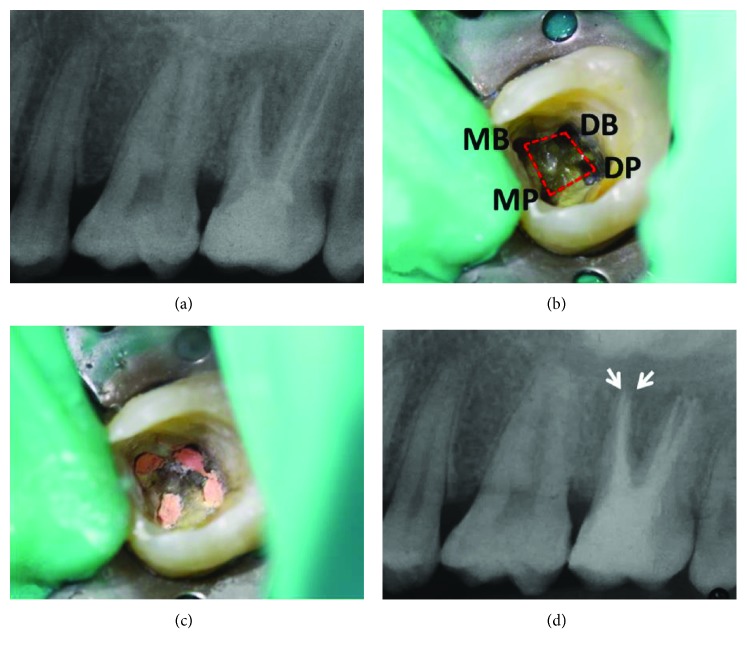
(a) The pretreatment radiograph showed an apical radiolucency on the mesial root. Due to the buccal-palatal superimposition, the second mesial root is not visible. (b) Photograph of the pulp chamber floor after removal of the gutta-percha. Root canal orifices were “irregular quadrilateral” shaped on the pulp chamber floor representing Versiani Type A. (c) Photograph after the canal obturation. (d) The posttreatment radiograph showed two mesial filled root canals (arrows).
